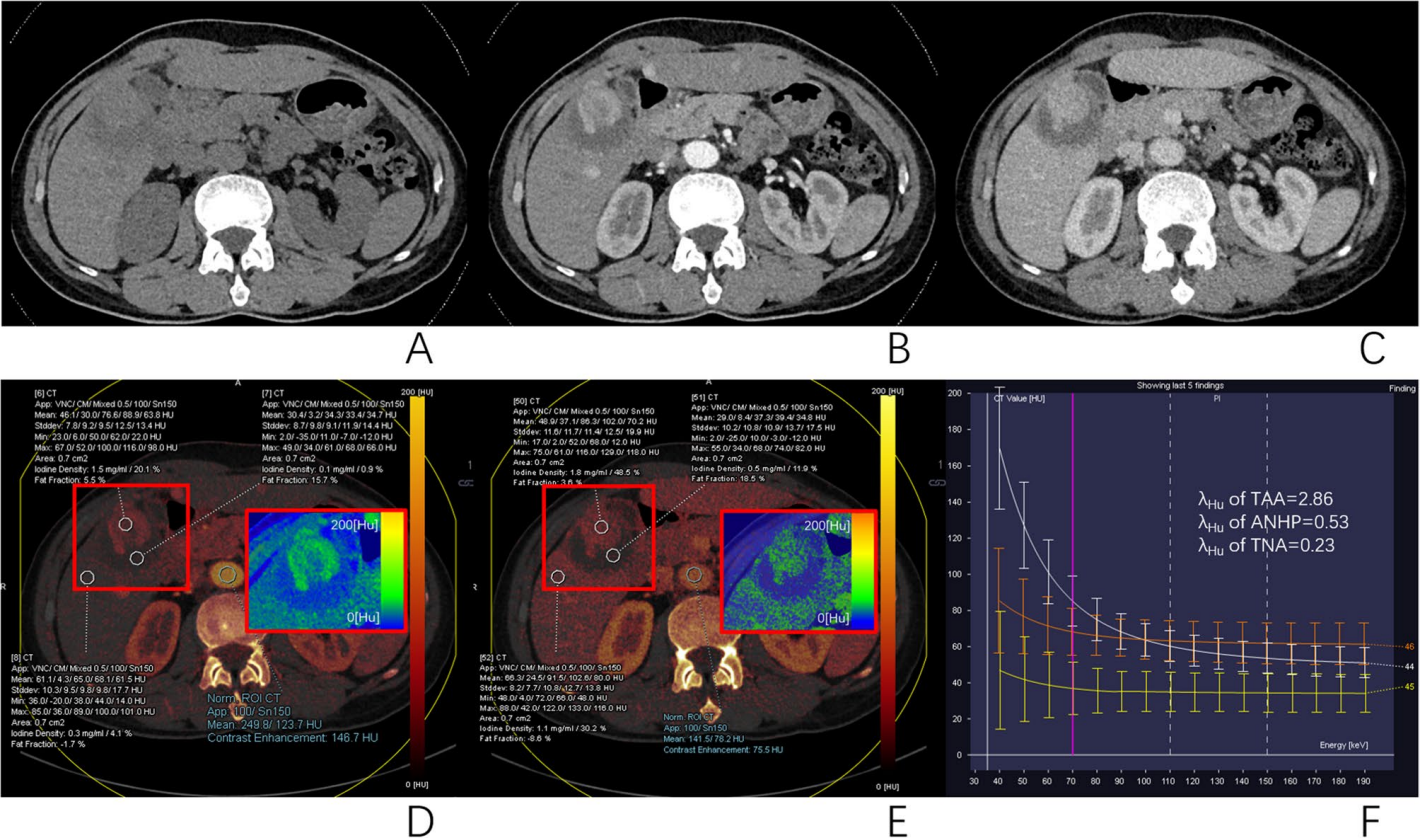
DECT images of a 58-year-old man with HCC undergoing DEM-TACE 6 weeks ago, showed that no lipiodol deposition in the original tumor lesions, but TAA and TNA were present. The right hepatic lobe showed a low-attenuation lesion on unenhanced CT image (A), significant enhancement at arterial phase (B), and persistent enhancement at the portal venous phase(C). TNA had no enhancement. ROIs of TAA, ANHP, and TNA placed on arterial and portal iodine map (D and E) showed IC and NIC of TAA to be 1.5 mg/ml and 20.1% at arterial phase and 1.8 mg/ml and 48.5% at portal venous phase, respectively. The IC and NIC of ANHP was 0.3 mg/ml and 4.1% at arterial phase, and 1.1 mg/ml and 30.2% at portal venous phase, respectively. The IC and NIC of TNA was 0.1 mg/ml and 0.9% at arterial phase and 0.5 mg/ml and 11.9% at portal venous phase, respectively. The λHu of TAA, ANHP and TNA were 2.86, 0.53 and 0.23, respectively (F).
